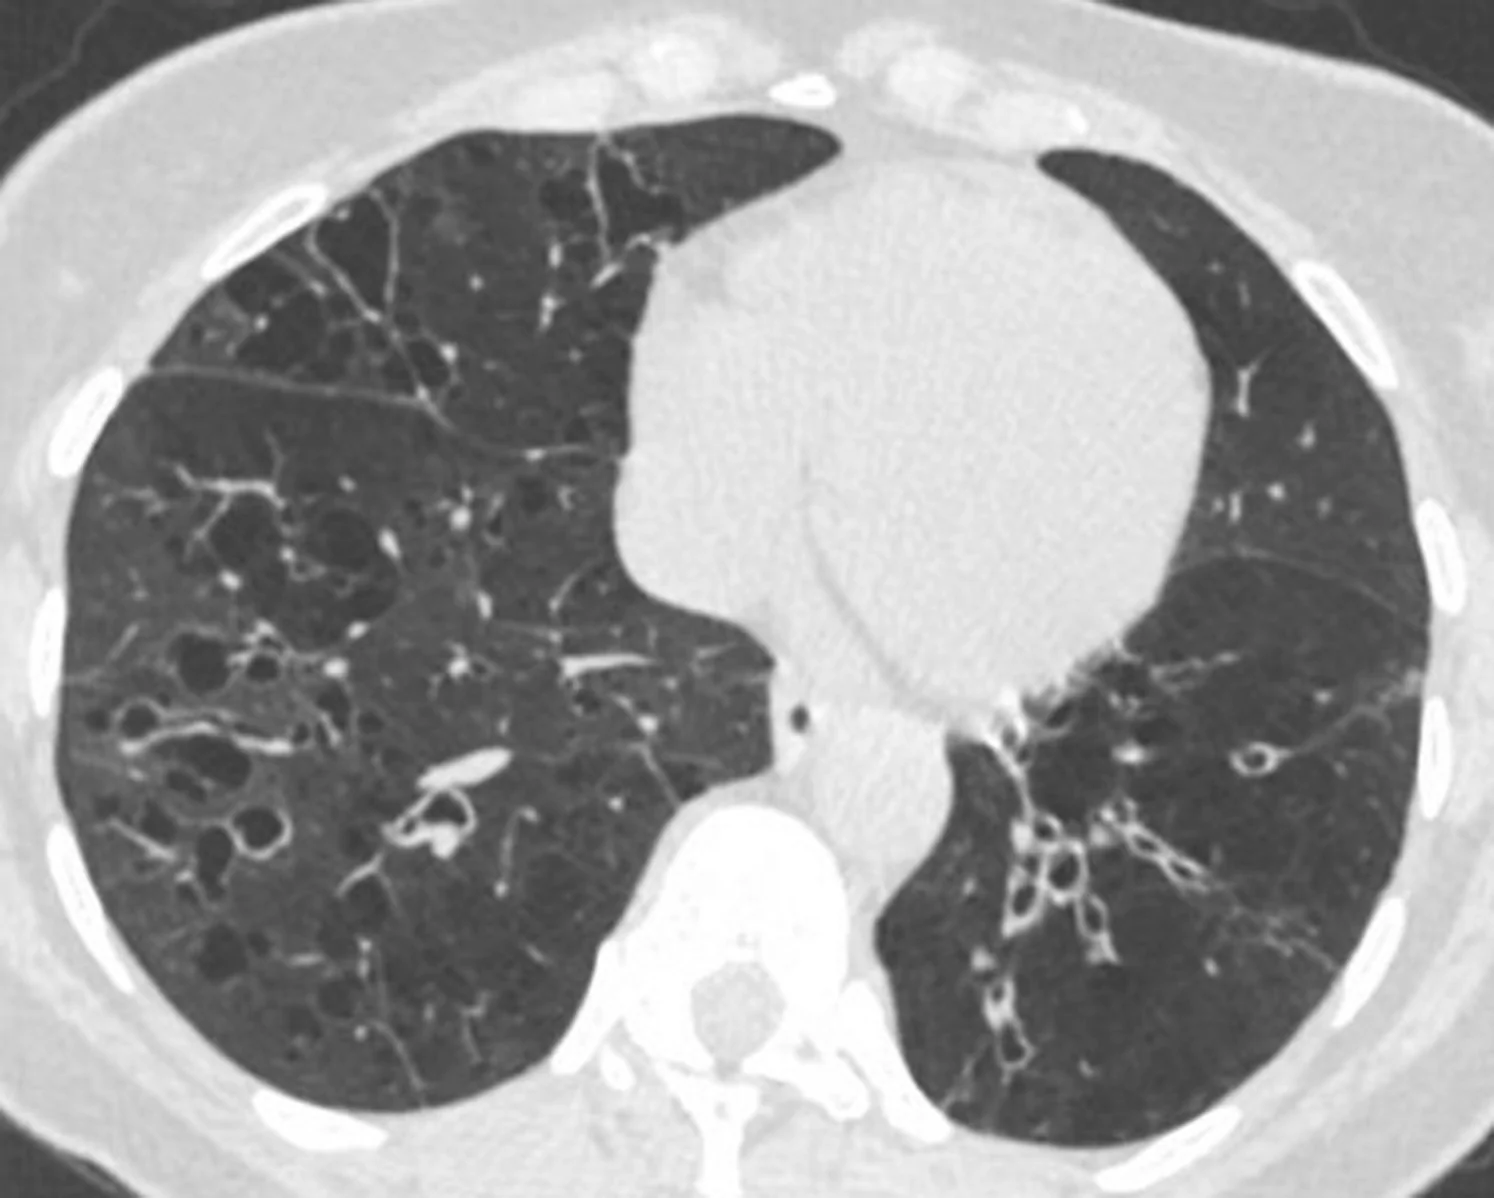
Axial CT scan of cylindrical basilar-predominant bilateral bronchiectasis.
